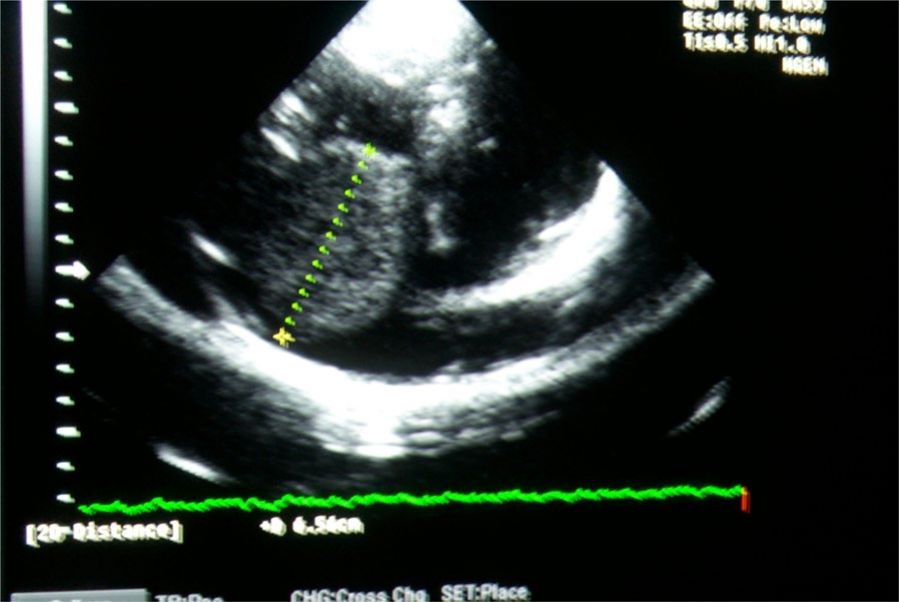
Appearance of the right atrial mass on transthoracic echocardiography
